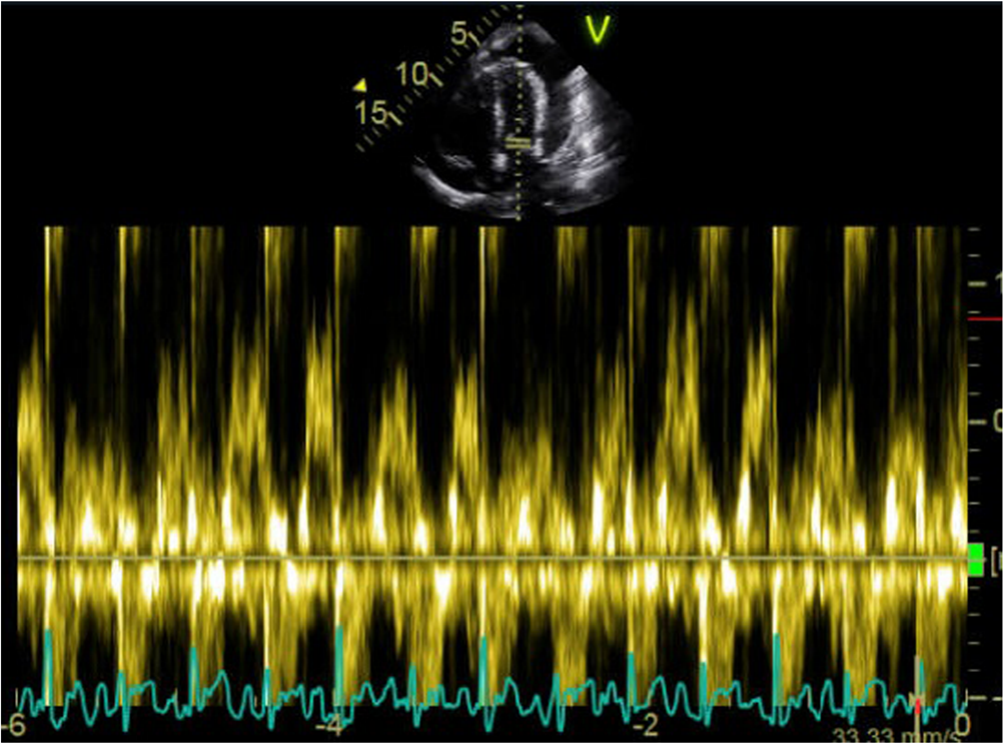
Transmitral Doppler profile demonstrating a > 25% variation in flow with respiration (indicative of tamponade physiology).
